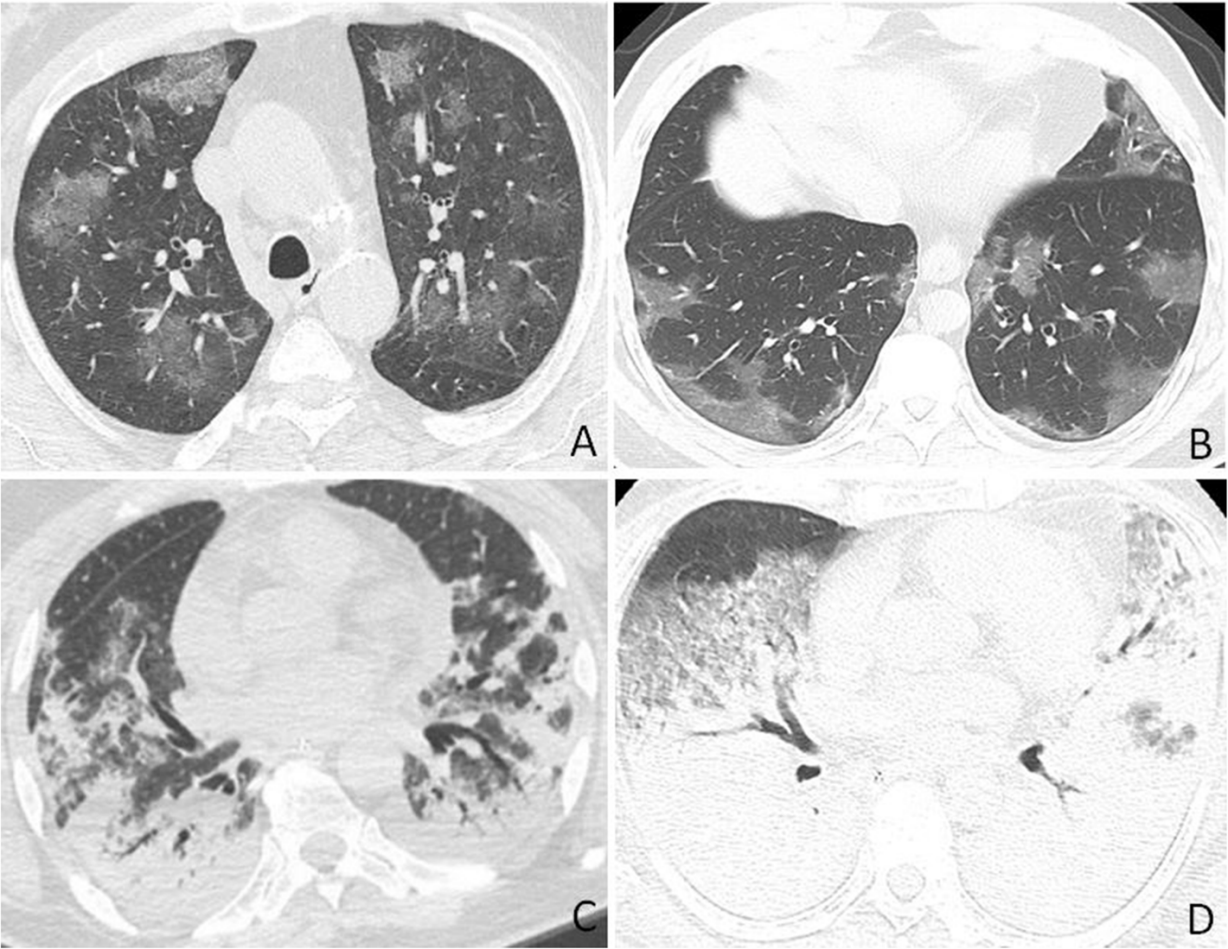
COVID-19 and bacterial pneumonia misdiagnosed as COVID-19. a Axial CT image obtained from a 49-year-old man with positive PCR test for COVID-19 shows peripherally located multifocal areas of GGO and superimposed interlobular septal thickening. b Axial CT image obtained for a 38-year-old man with Klebsiella pneumonia shows peripherally located GGO as shown in (a). c Axial CT image obtained from a 67-year-old woman admitted to ICU with positive PCR for COVID-19 and secondary infection by *Staphylococcus aureus*; axial CT shows bilateral consolidation with small areas of GGO and bronchial dilatation. d Axial CT image from a 72-year-old woman with MRSA pneumonia (Methicillin-resistant *Staphylococcus aureus*) shows bilateral larger consolidations and GGO than the ones seen on (c)
